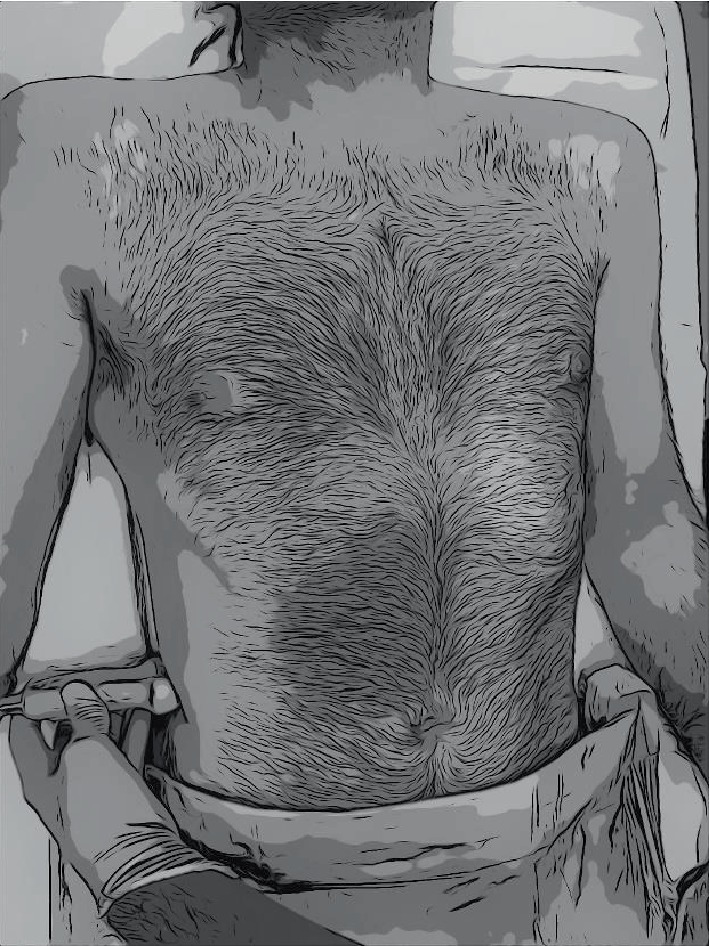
Placement of linear ultrasound transducer on the posterior aspect to allow visualization of posterior aponeurosis of the transversus abdominis muscle.
